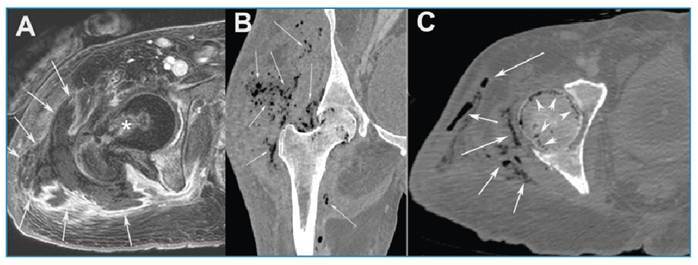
A fat-suppressed T1-weighted MR image acquired after intravenous contrast administration (A) reveals inflammatory changes in the periarticular soft tissues and abscessed areas (arrows). The bone marrow of the proximal femur and acetabulum shows mild enhancement (asterisk). Coronal (B) and axial (C) non-enhanced CT images (bone window) demonstrating multiple small foci of intramedullary gas in the right femoral head and neck (arrowheads), along with soft-tissue emphysema (arrows).
